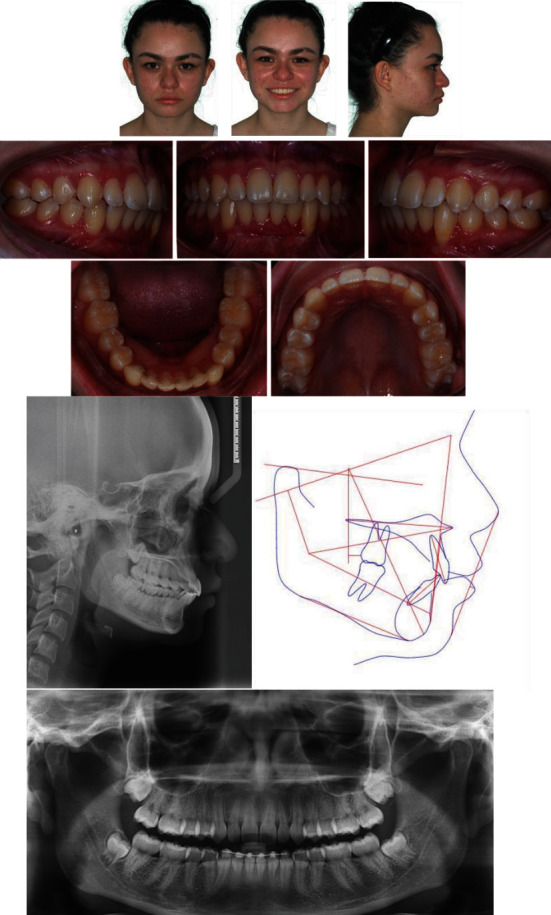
Extra-oral and intra-oral photographs and final radiographic-cephalometric records.
